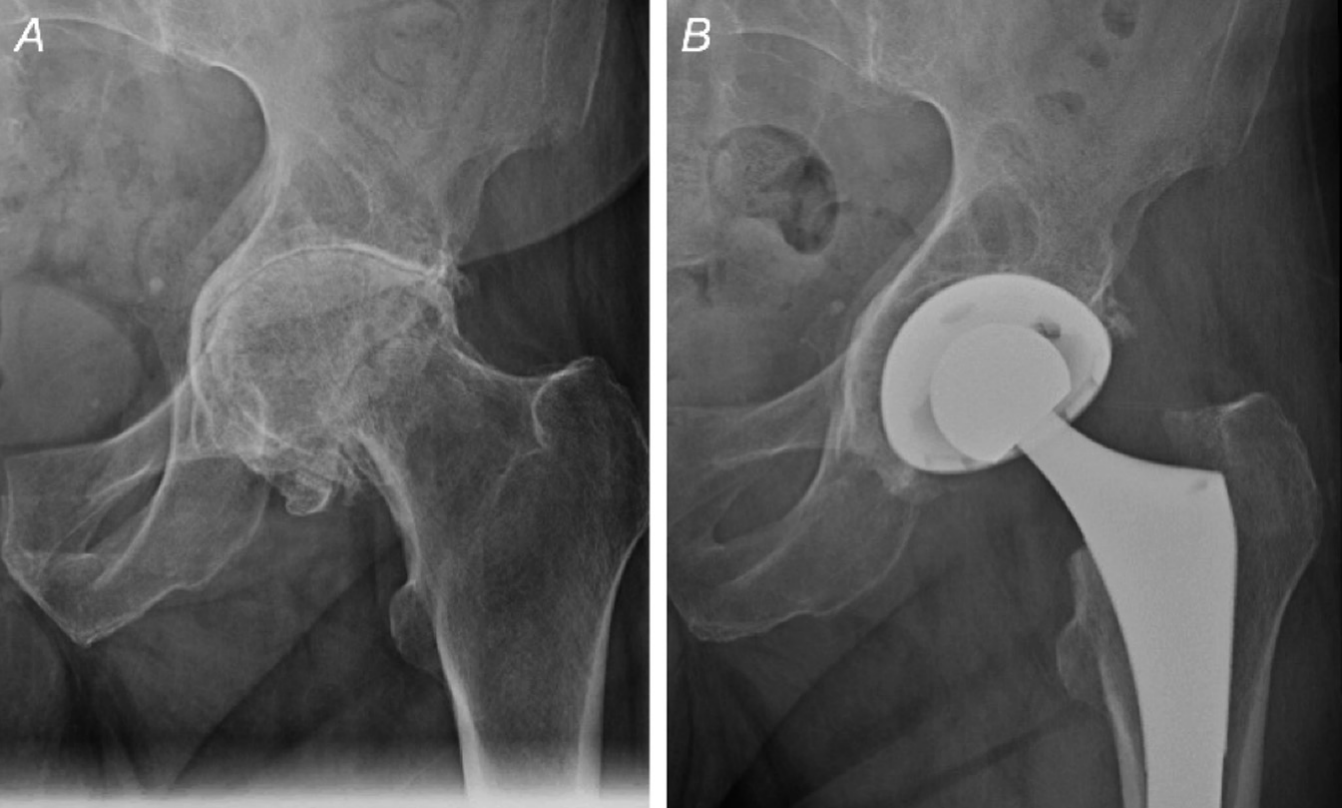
A and B, Radiographs of the hip demonstrating acetabular protrusio (A) in a patient who was treated with total hip arthroplasty (B).
